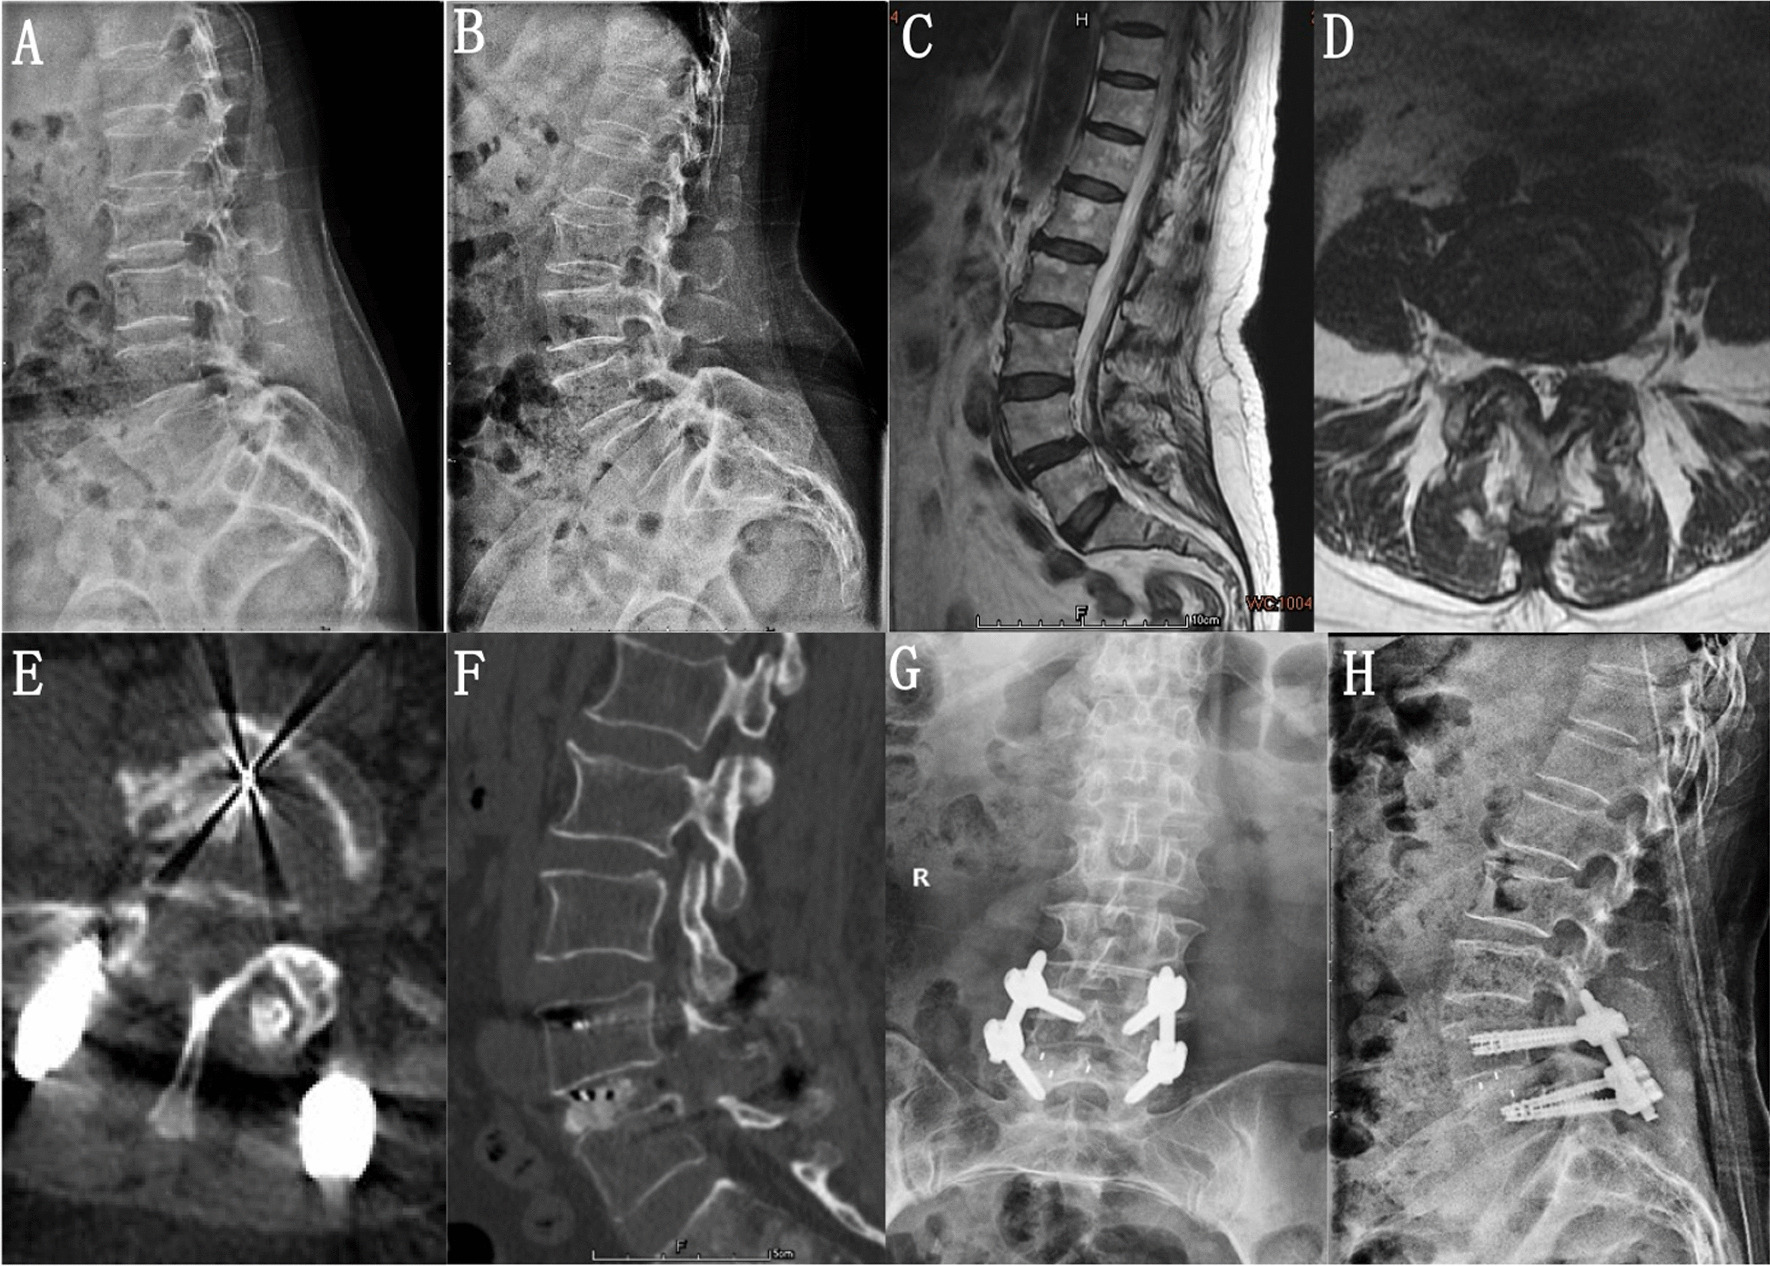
Case of a 65-year-old female patient who underwent unilateral biportal endoscopy lumbar interbody fusion (UBE-TLIF). A, B Preoperative dynamic radiography showed spondylolisthesis at the L4–5 level. C, D Preoperative magnetic resonance imaging (MRI) showed lumbar spinal stenosis at the L4–5 level. E Postoperative computed tomography (CT) showed unilateral laminectomy. F Postoperative CT showed that the cage position was good. G, H The last follow-up showed bone fusion between the vertebral bodies
